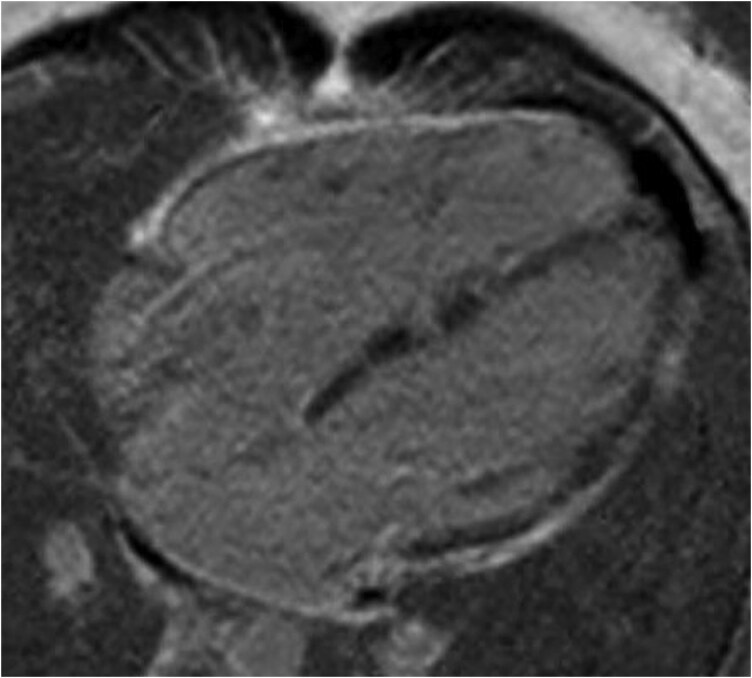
A cardiac MRI showing an enlarged right ventricle with an end-diastolic-volume-index of 151 ml/m^2^ (upper limit of normal 87 ml/m^2^) and a calculated RVEF of 30%. There was late gadolinium enhancement in the right and left ventricular walls, suggesting fibrofatty infiltration. The sequence shown is delayed enhancement inversion recovery T1. This figure shows a clear suggestion of arrhythmogenic cardiomyopathy.
